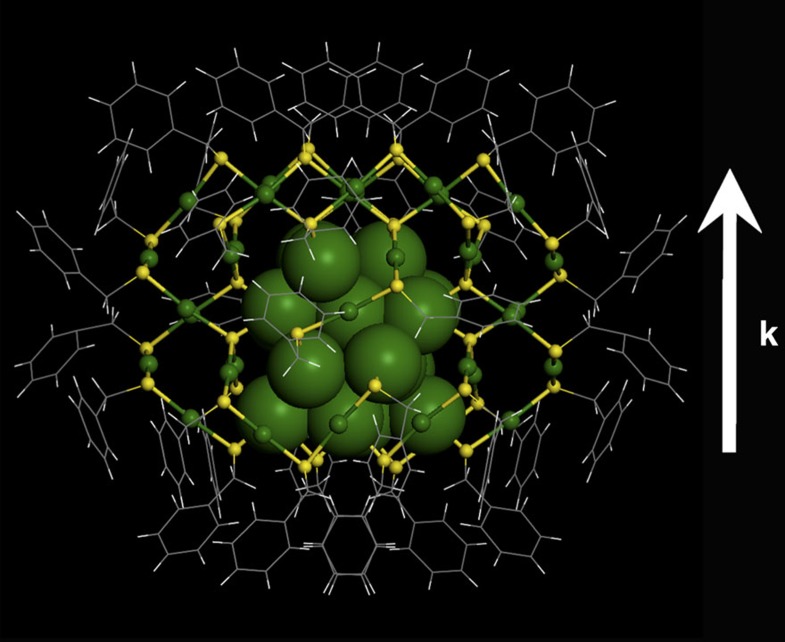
X-ray atomic structure of the Au_60_S_6_(SCH_2_Ph)_36_ nanocluster. The Au atoms are in green, the sulfur atoms are in yellow, and the benzyls are in wireframe. The vertical upward arrow indicates the direction of the k-vector.
